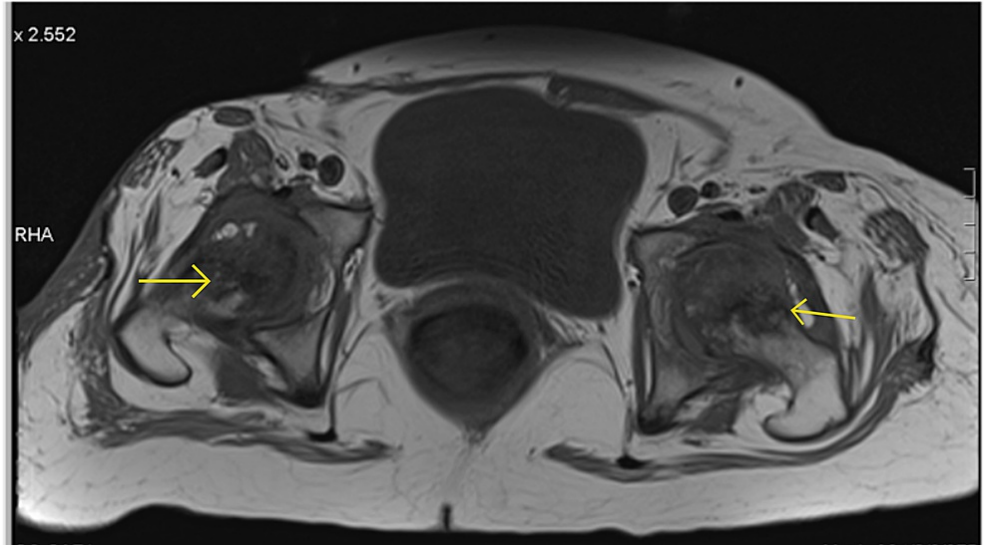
Pelvis MRI axial view Yellow arrows show bilateral sepiginous low-signal circumscribed areas in both femoral heads.
